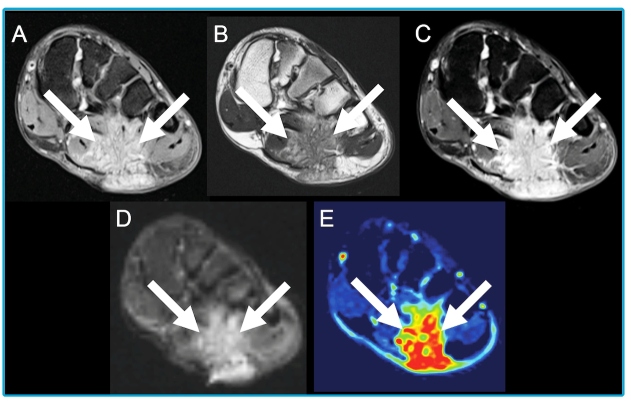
MRI of the left foot showing a large mass comprising multiple tiny clustered oval lesions affecting the left foot plantar soft tissues and infiltrating the flexor digitorum brevis and flexor hallucis brevis muscles and plantar fascia. The mass had a predominantly isointense signal on T1-weighted image with fat saturation (arrows in A) and hypointense signal on the T2-weighted image (arrows in B) due to the associated surrounding fibrous reaction; it showed heterogeneous gadolinium-enhancement (arrows in C). The lesion also presented restricted diffusion due to hypercellularity (arrows in D), and increased perfusion in its solid portions (arrows in E).
